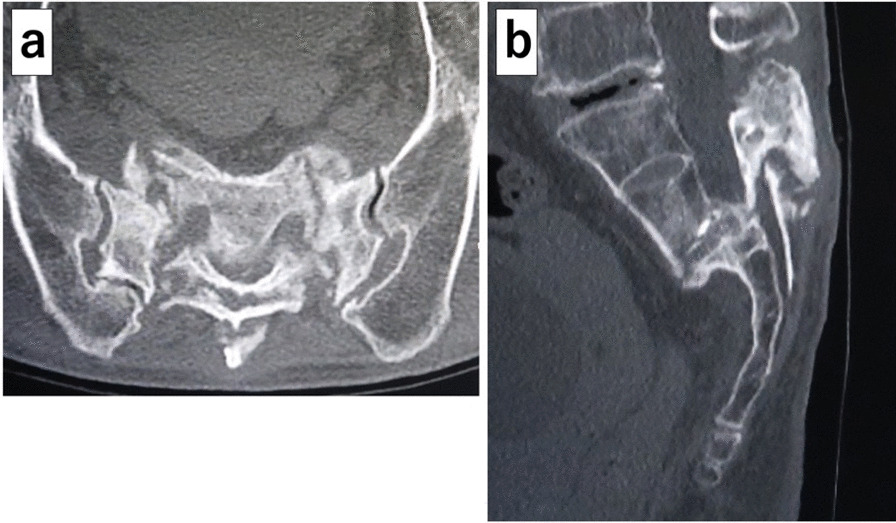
Preoperative computed tomography. a Sagittal reconstruction image showing the sacral fracture consisting of a transverse fracture with displacement of the third sacral vertebra. b Axial image showing vertical fractures of the bilateral sacral wings
